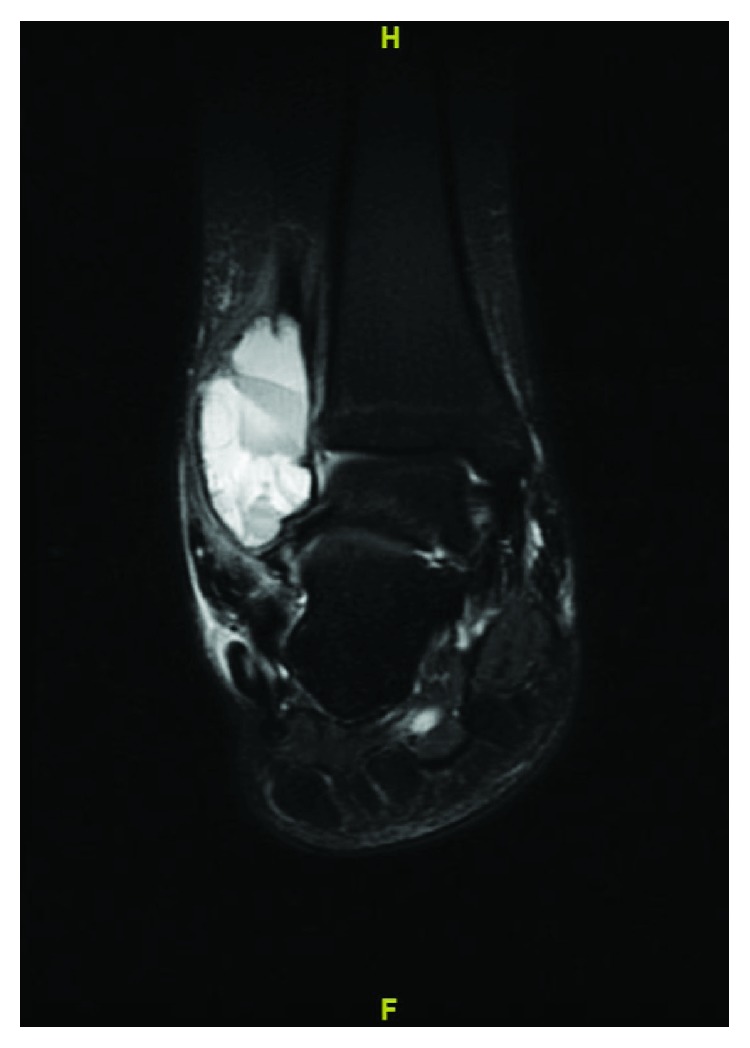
STIR (short tau inversion recovery) weighted MRI of the ankle showing 5 × 5.2 × 3.6 cm multiloculated, expansile lesion with fluid-fluid levels.
